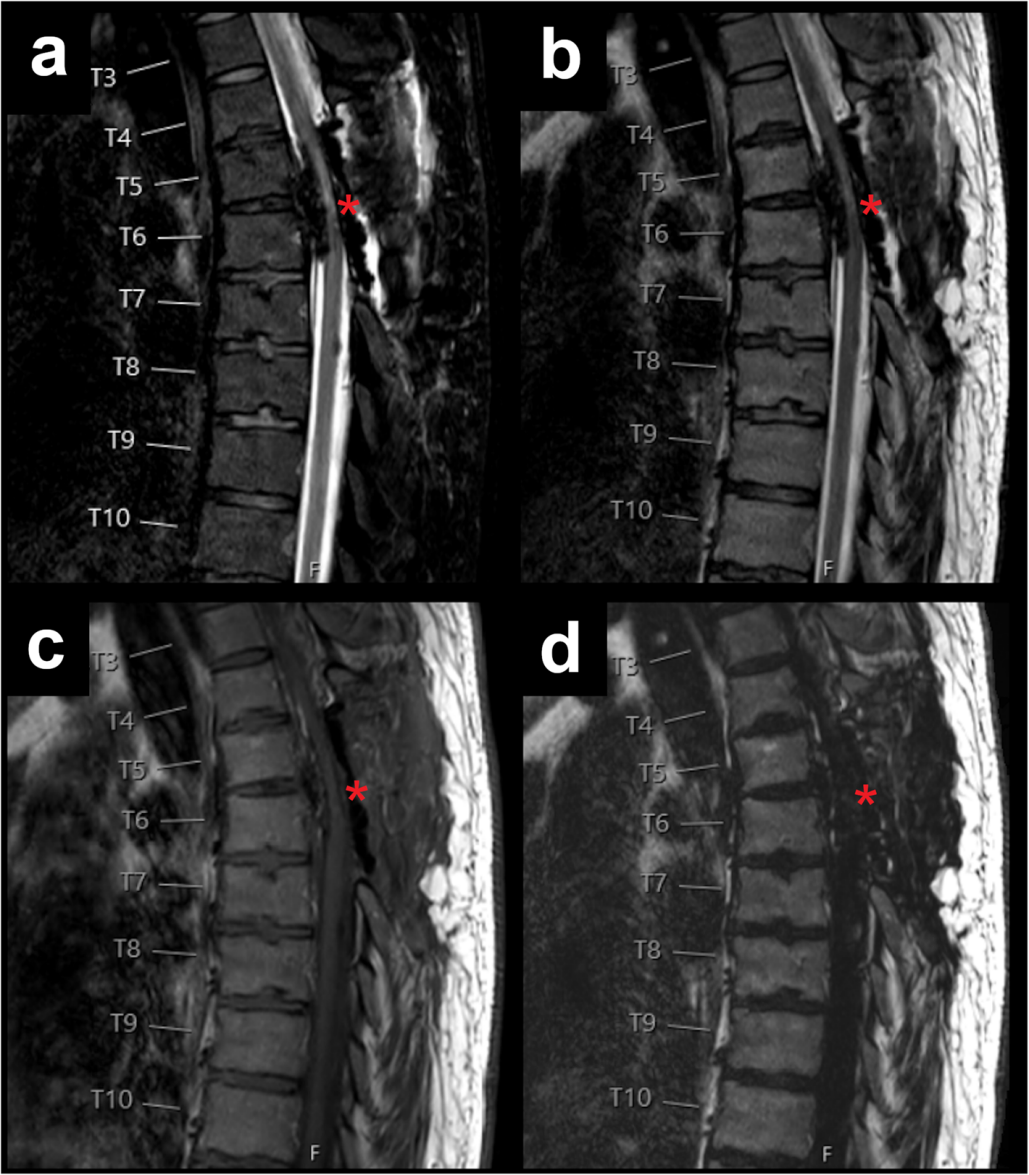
Postoperative thoracic haematoma. This figure shows the thoracic spine of a 39-year-old female patient using water-only images (a), in-phase images (b), and fat-only images (d) of the sagittal T2-weighted DIXON sequence, together with a sagittal T1-weighted sequence (c). The patient had undergone decompressive thoracic surgery and presented with a postoperative intradural extramedullary haematoma between T4 and T7 (red asterisks)
